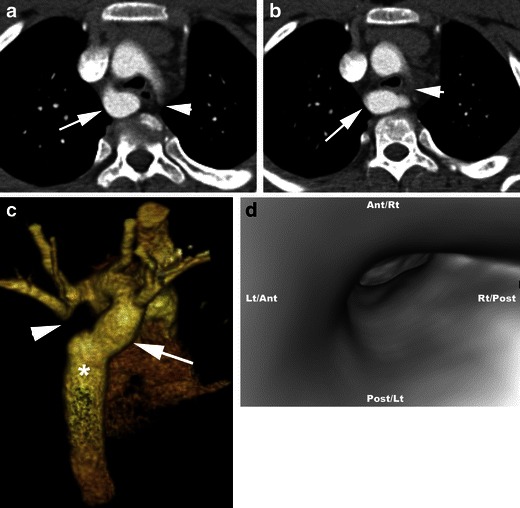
Double aortic arch with an atretic left arch. a and b Axial CT scan images show bilateral aortic arches. The right aortic arch is dominant (*arrow*). The left aortic arch is atretic (*arrowhead*). c 3D volume-rendered image demonstrates the double aortic arch. The dominant right aortic arch (*arrow*) gives off the right subclavian and right common carotid branches, while the left atretic aortic arch gives off the left common carotid and left subclavian arteries proximal to the atretic region (*arrowhead*). Only the right aortic arch joins the descending aorta (*). d Virtual bronchoscopy demonstrates severe narrowing of the airway by the double aortic arch with atretic left arch
